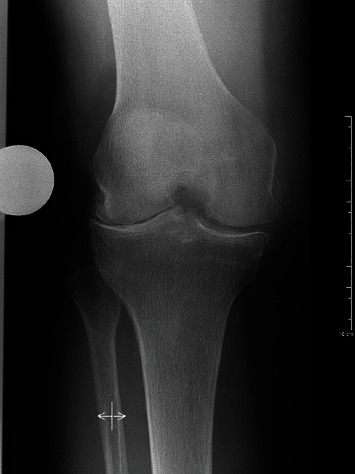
Preoperative radiographs showing the anteroposterior view of the right knee.
